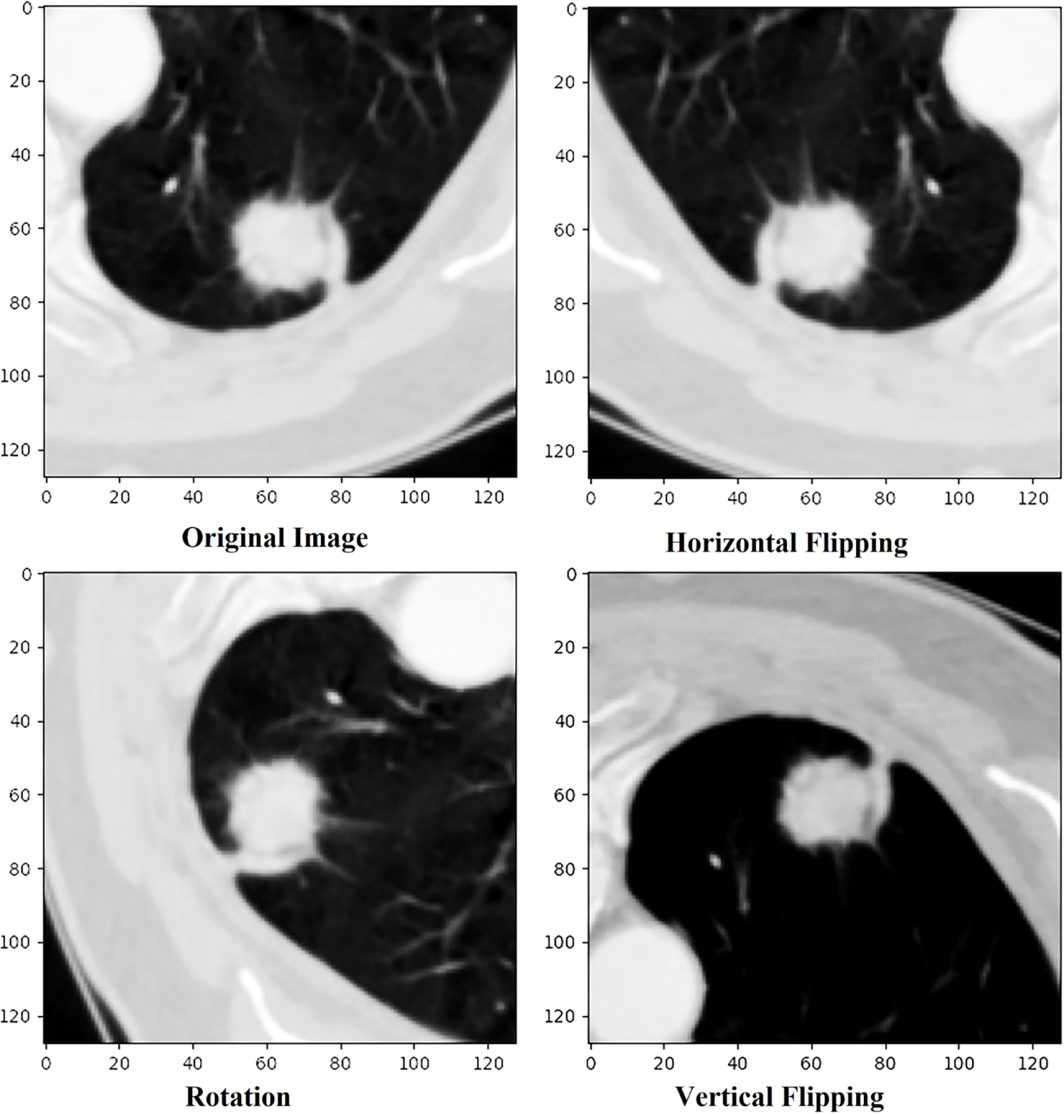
Original CT slice with three variants of data augmentation techniques.
